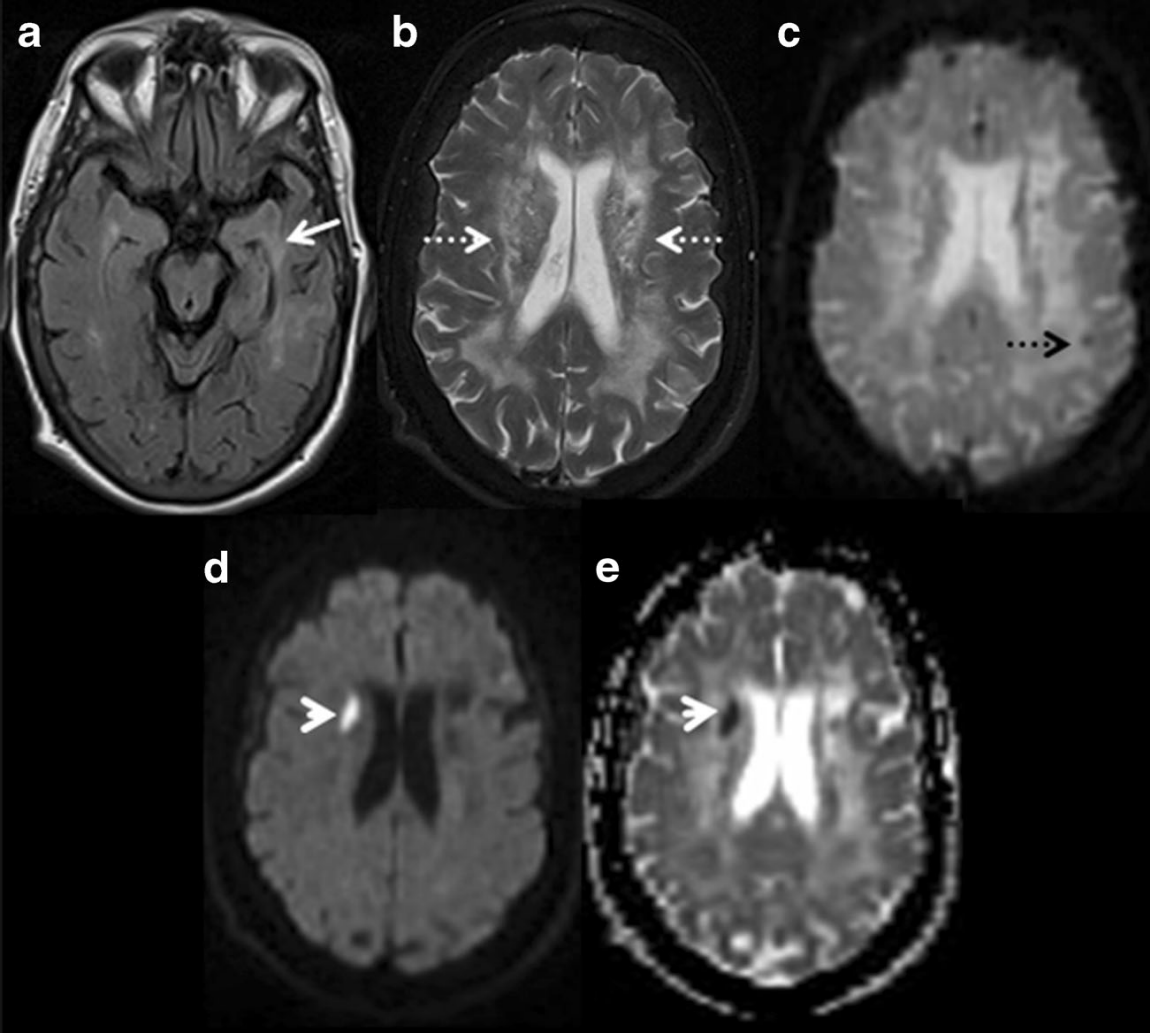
CADASIL. A 51-year-old female with left hand weakness, slurred speech and dysarthria with progressive cognitive decline. a & b axial FLAIR and T2W images of brain demonstrate patchy confluent hyperintensities in periventricular (*white dashed arrows*) and subcortical white matter with typical involvement of temporal lobes (*white arrow*). c axial GRE image shows presence of microhaemorrhage in the subcortical white matter (black dotted arrow). d & e axial DWI and ADC map of brain reveal focal area of restriction of diffusion (*white arrow head*) in the right corona radiata, suggesting acute infarct
